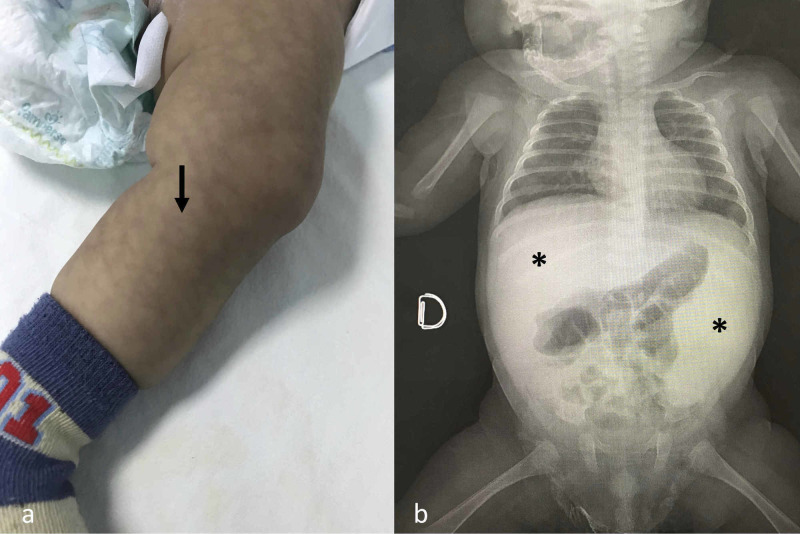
The patient on admission to the community hospital had (a) mottled skin with reticular aspect (arrow) and (b) severe hepatosplenomegaly also visible on abdominal plain X-ray film (asterisks).
